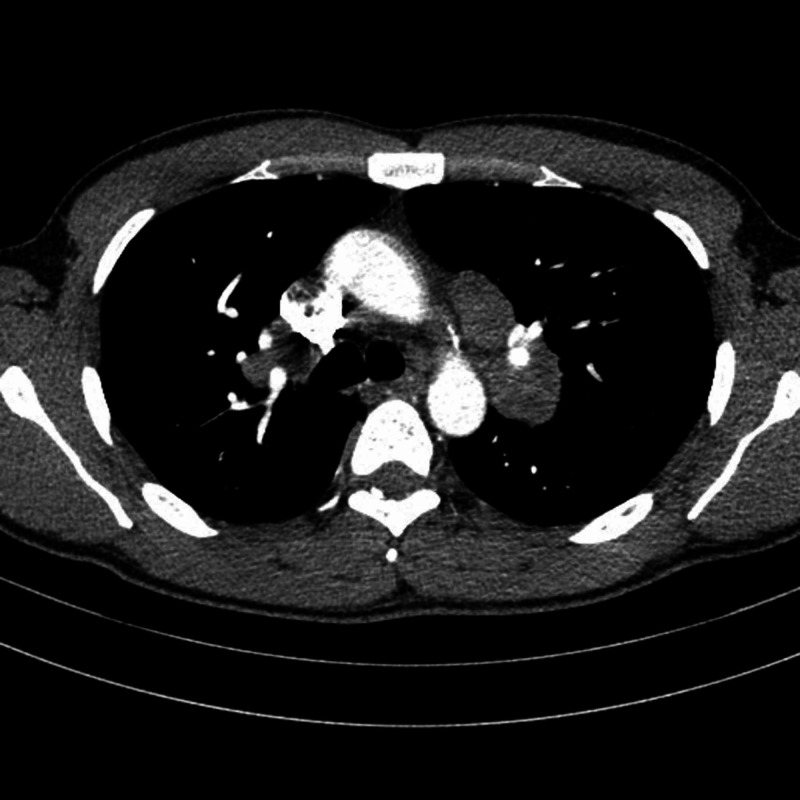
CT thorax revealing bilateral hilar lymphadenopathy
